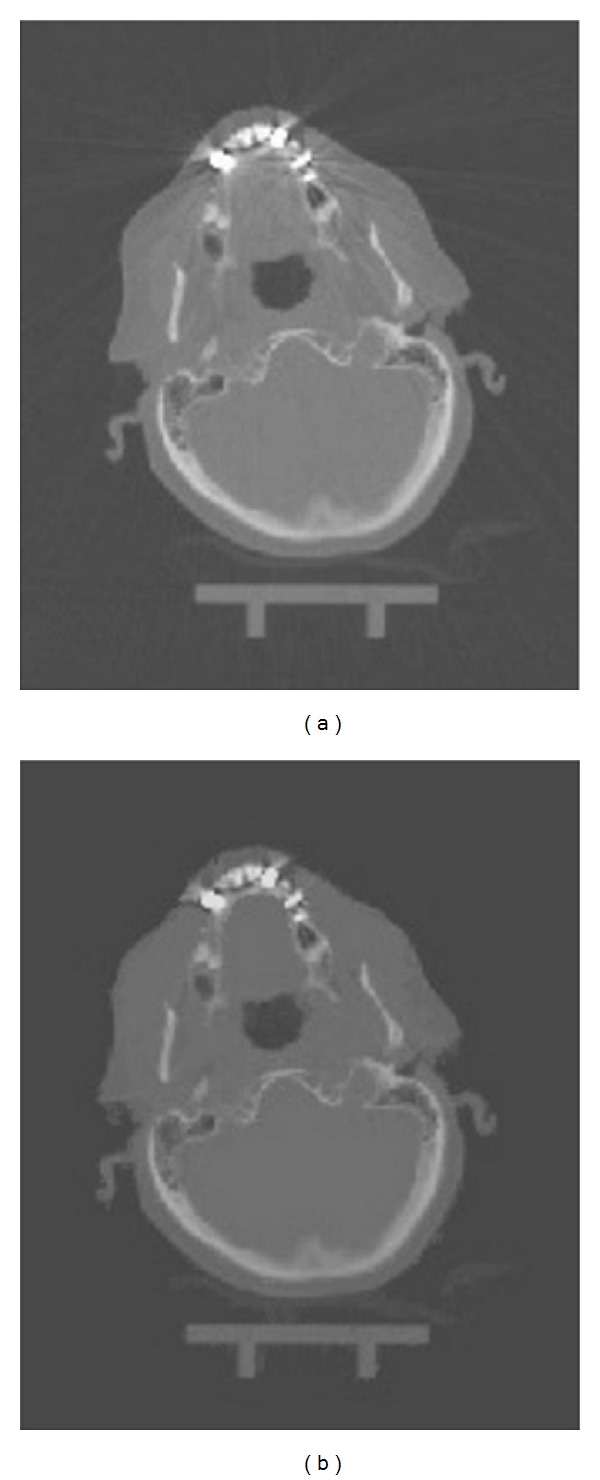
Axial view of CT dataset before (a) and after correction (b).
